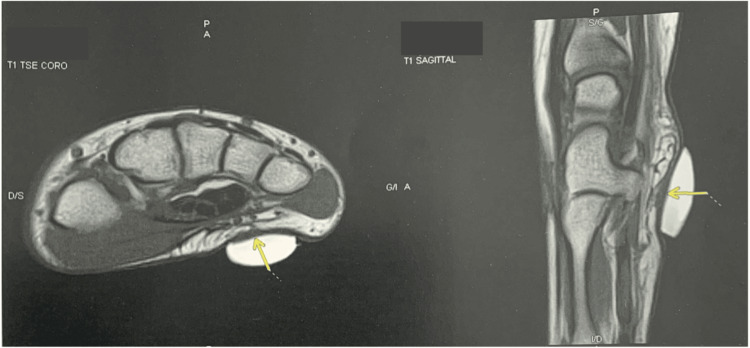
Two-month postoperative MRI The yellow arrow indicates scar tissue measuring 10 × 6 × 3 mm
